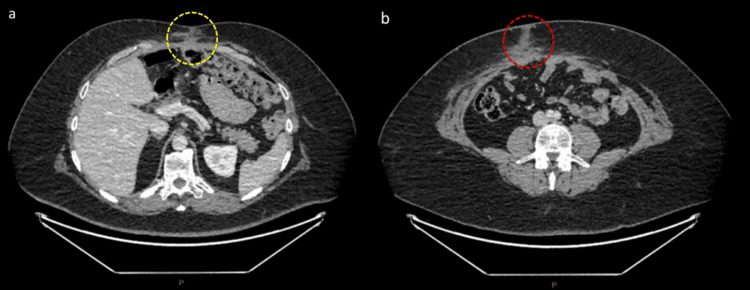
CT abdomen portal venous phase five months post-surgery a: Axial slice with 0.625 mm thickness, W:400/L:40, demonstrating no evidence of recurrence at the superior tumour site (yellow dotted oval). b: Axial slice with 0.625 mm thickness, W:400/L:40, demonstrating no evidence of recurrence at the inferior tumour site (red dotted oval).
